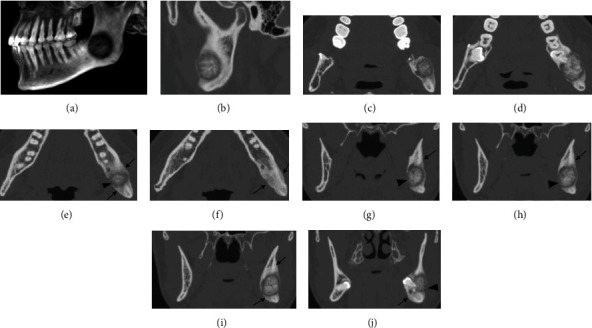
Case #1 3D CT reconstruction (a) and bone window CT sagittal view (b) showing the mixed density lesion associated with impacted #17 measuring 1.6 × 2.1 × 2 cm. The border of the lesion is well circumscribed with a broad sclerotic rim surrounding a lucent halo. Axial views ((c-f) from superior to inferior) and coronal views ((g-j) from posterior to anterior) showing buccal and lingual expansion, thinning, and areas of perforation at buccal and lingual cortices (arrowheads). Extensive sclerotic reactive bone is seen surrounding the lesion. New bone formation can be seen at buccal, lingual cortical, and inferior to the lesion (arrows). The lucent rim of the lesion extends into the left mandibular canal, with potential involvement of the inferior alveolar nerve (g–j).
